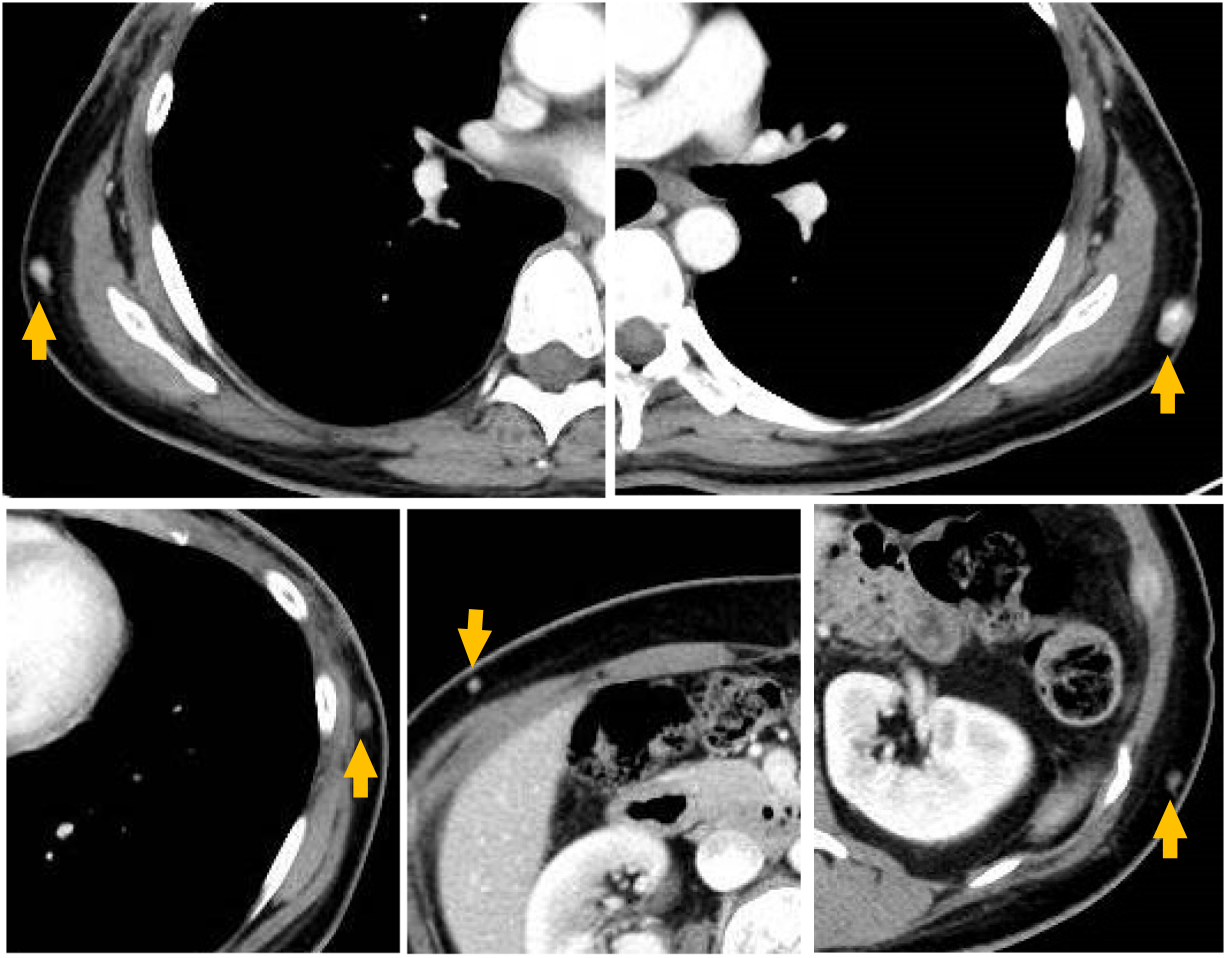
Before adjuvant chemotherapy, 11 weeks after the surgery, computed tomography revealed multiple subcutaneous small nodules on the chest wall outside the irradiation field (arrows). These were suspected of being angiosarcoma metastases
